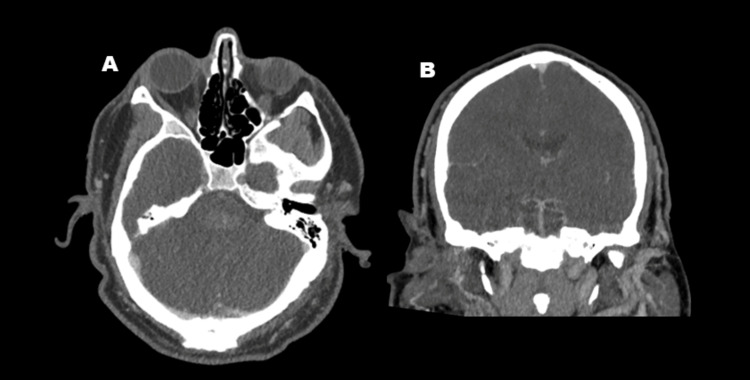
No vascular abnormalities in posterior circulation. The axial (A) and coronal (B) of CT angiogram showed no vascular malformations or stenosis.
